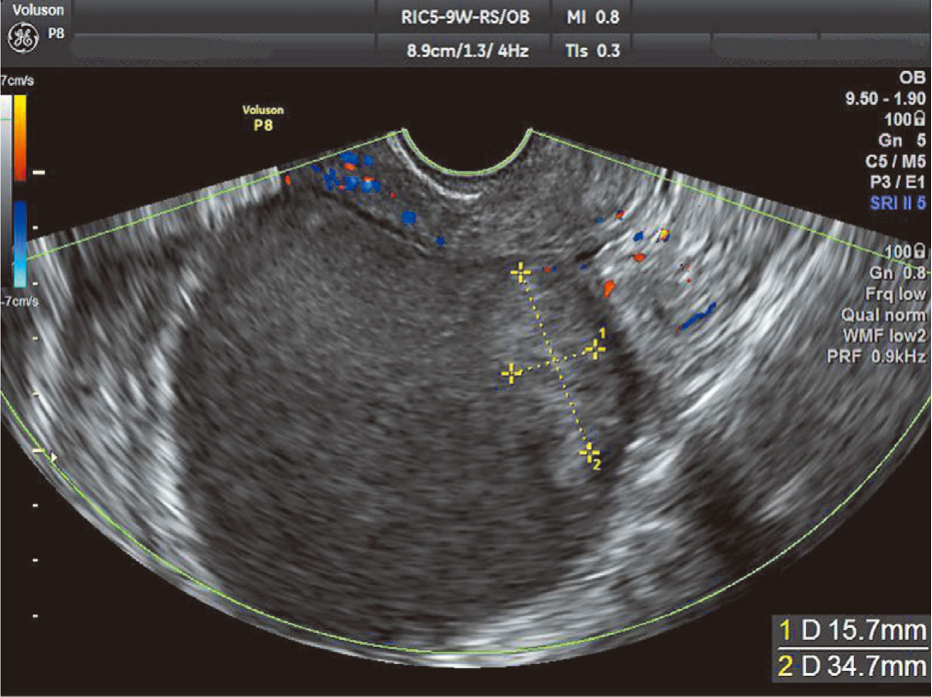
Transvaginal ultrasound sagittal plane image. Color Doppler enables confirmation that sites with solid echoes have no blood flow.
